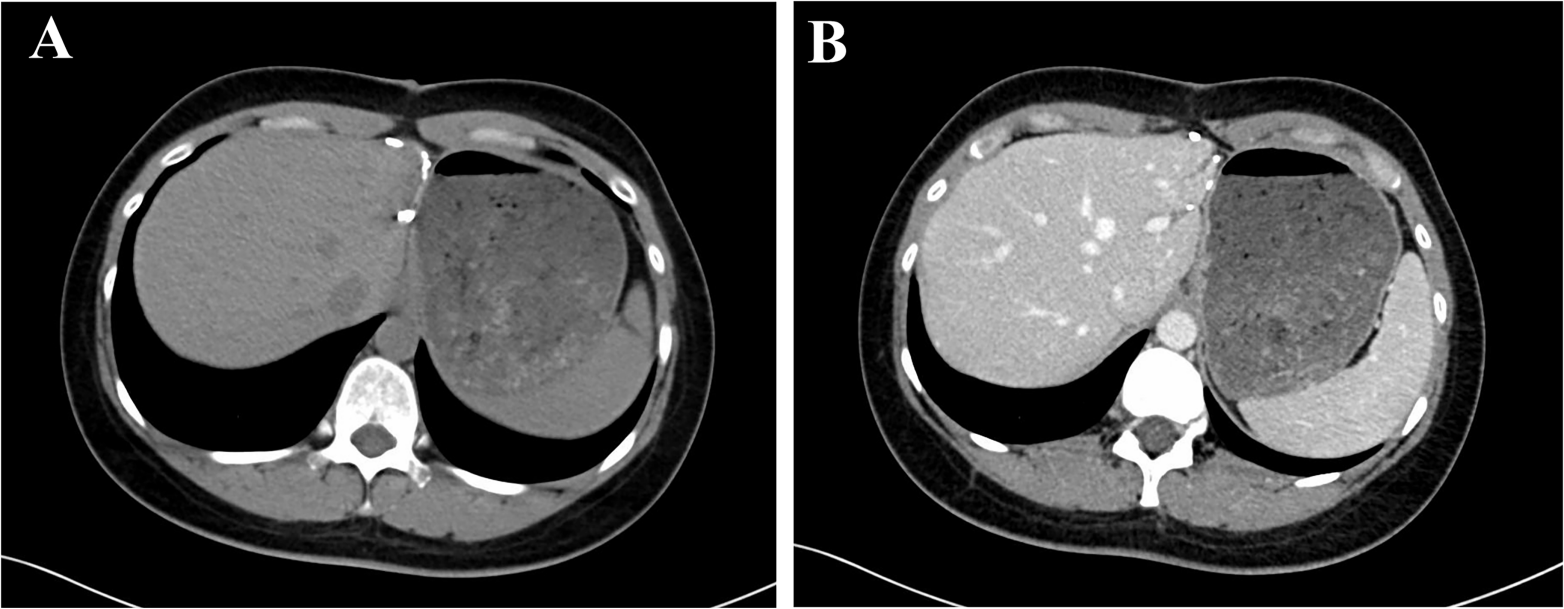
CT showed the patient was free from tumor recurrence after a 28 months follow-up.
